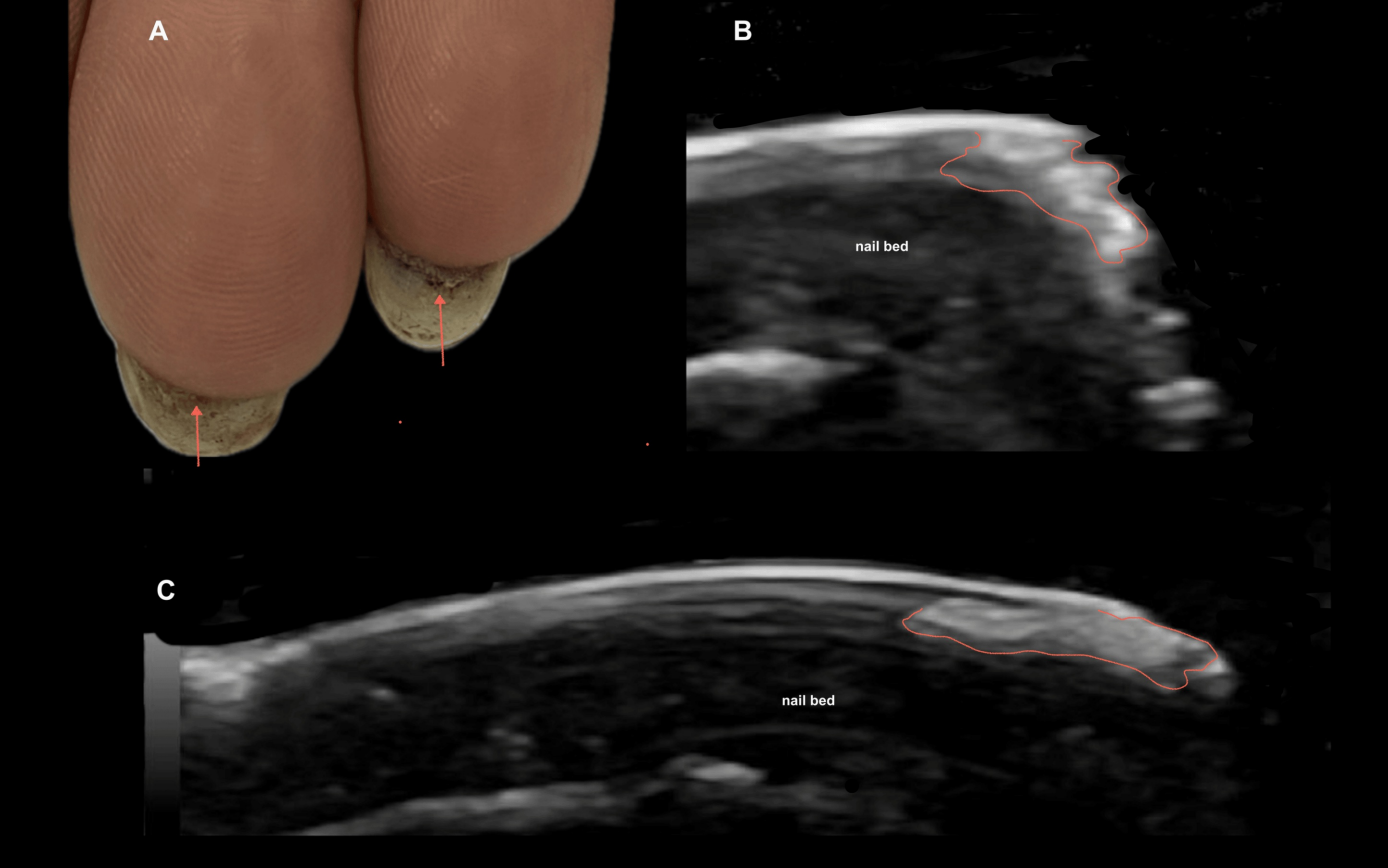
Pterygium inversum unguis in a gel polish user: clinical and ultrasonographic correlation. Female patient of 19 years of age permanent user of gel nail polish. (A) Clinical image showing pterygium inversum unguis (PIU) indicated by red arrows on the third and fourth fingers. (B) Magnified longitudinal grayscale ultrasound image of the distal third of the third ungual unit, delimited in red, showing defined, irregular hyperechoic tissue corresponding to PIU, where the hyponychium adheres to the ventral nail plate. (C) Longitudinal ultrasound image of the fourth ungual unit, also delimited in red, demonstrating hyperechoic tissue consistent with PIU, which appears more longitudinal in this finger. Ultrasound allows a precise definition of the extent of PIU.
